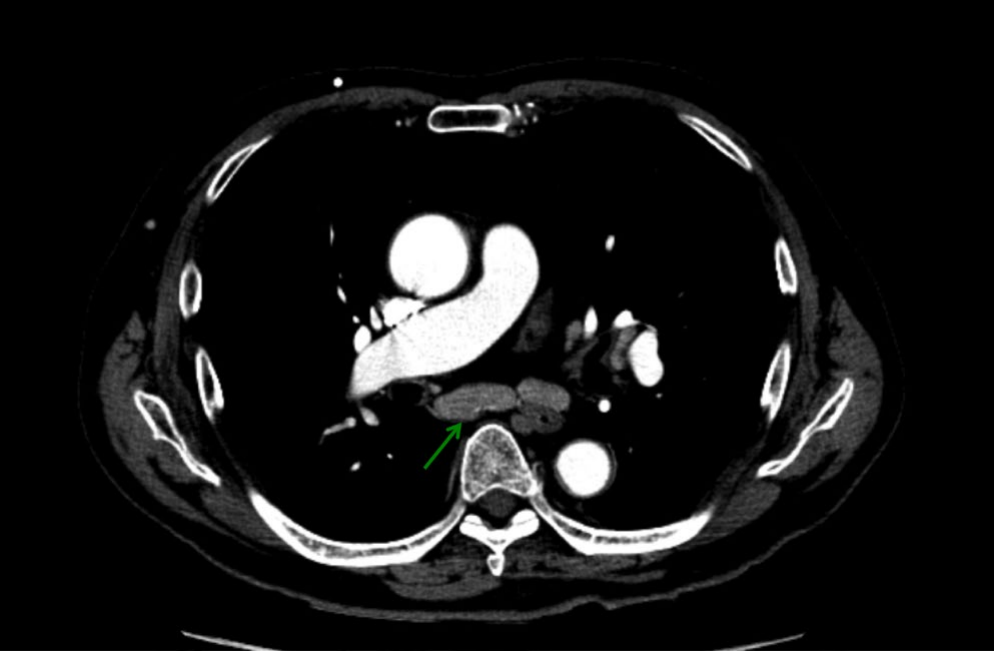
Post‐contrast axial chest computed tomography. Cardiovascular abnormalities are not observed. The subcarinal lymph nodes (green arrow) are slightly enlarged
